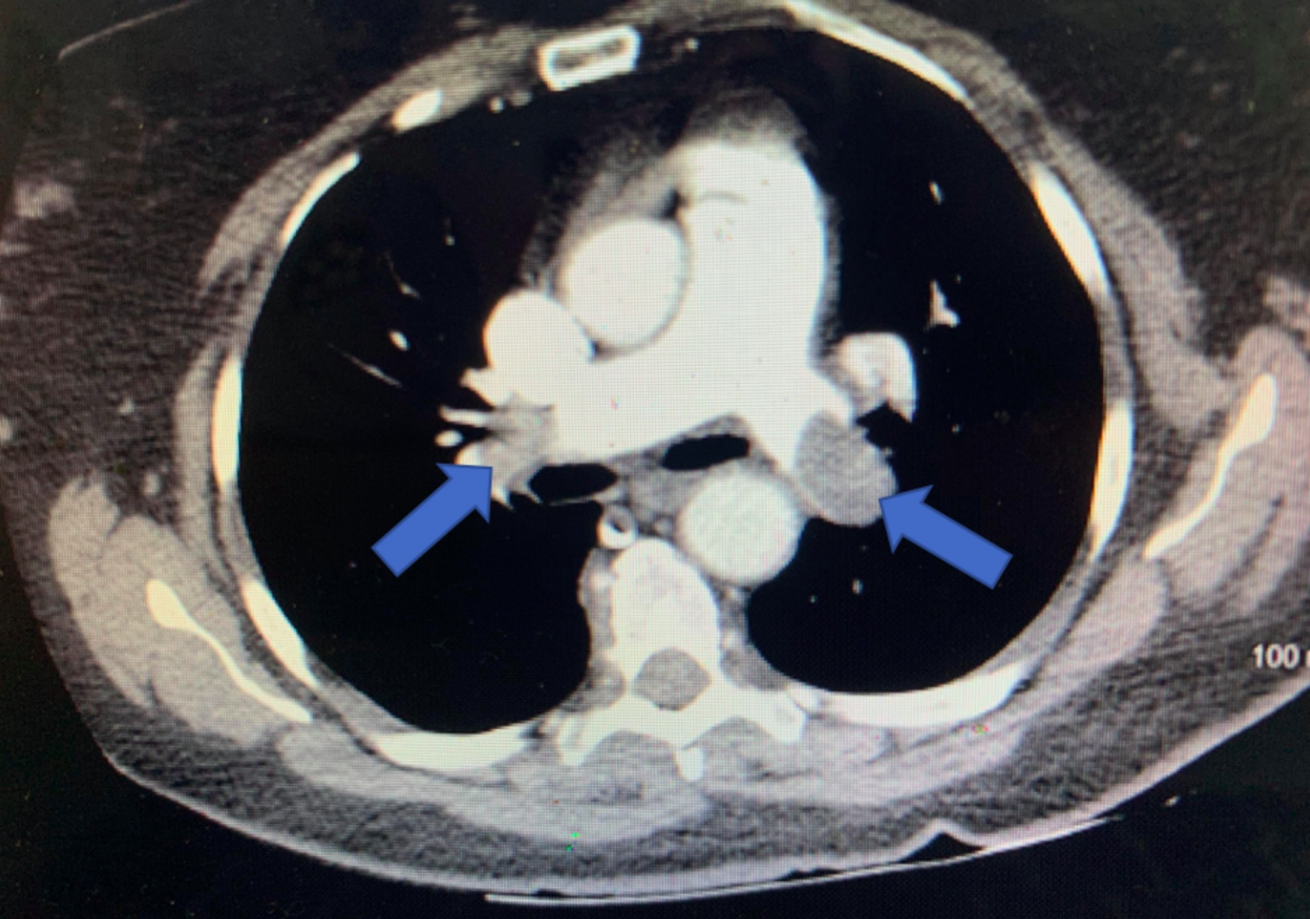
CT angiography showed prominent bilateral pulmonary emboli
